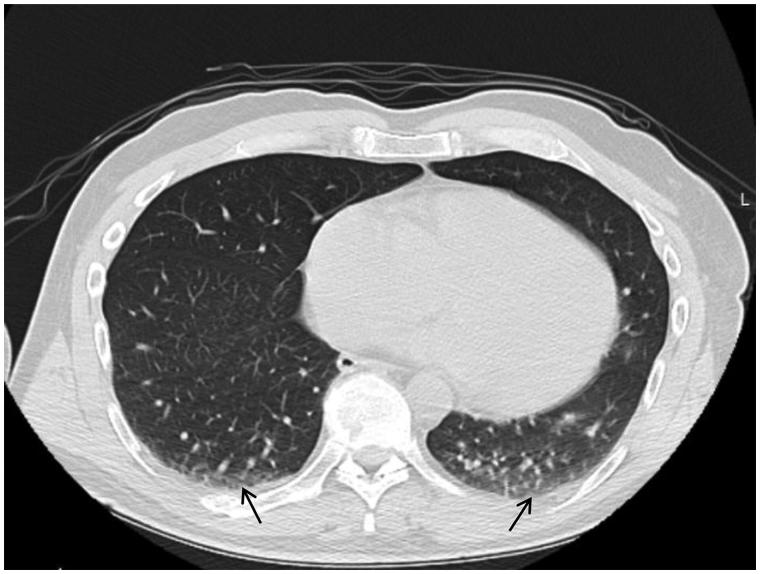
CT scan of one of the study subjects at the end of surgery. The arrows indicate small areas of atelectasis formation. In this case, 1.9% of the total lung area was affected, and this value corresponded to the median of all subjects in the study.
